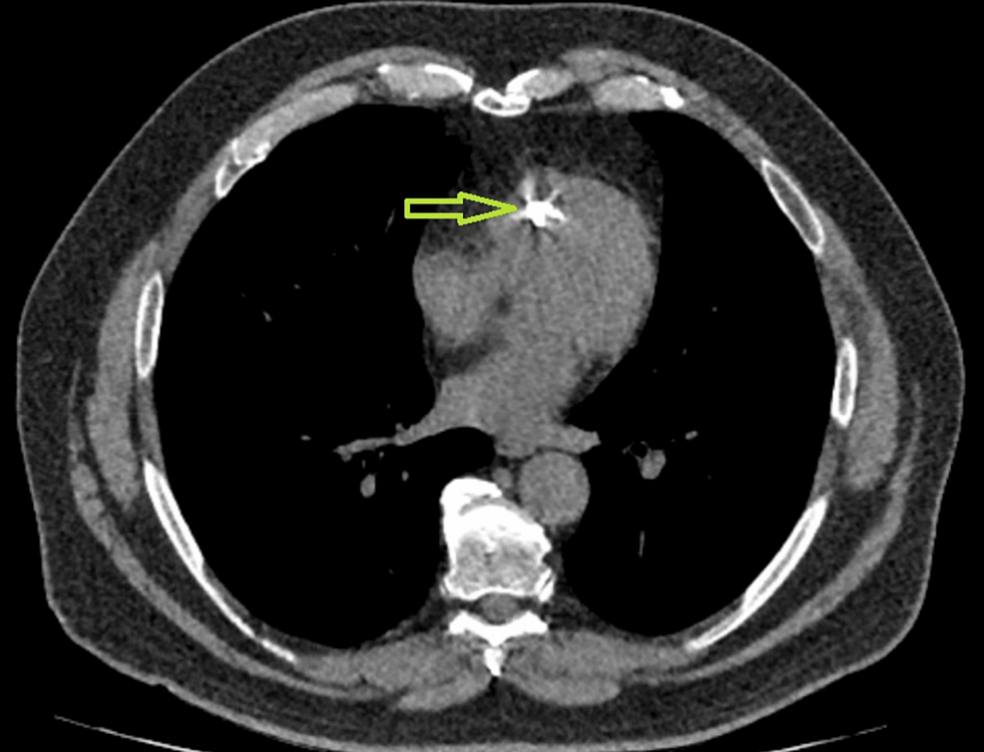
An axial section of the non-contrast CT chest, with an arrow pointing to the dense mass attached to the interventricular septum. CT, computed tomography
